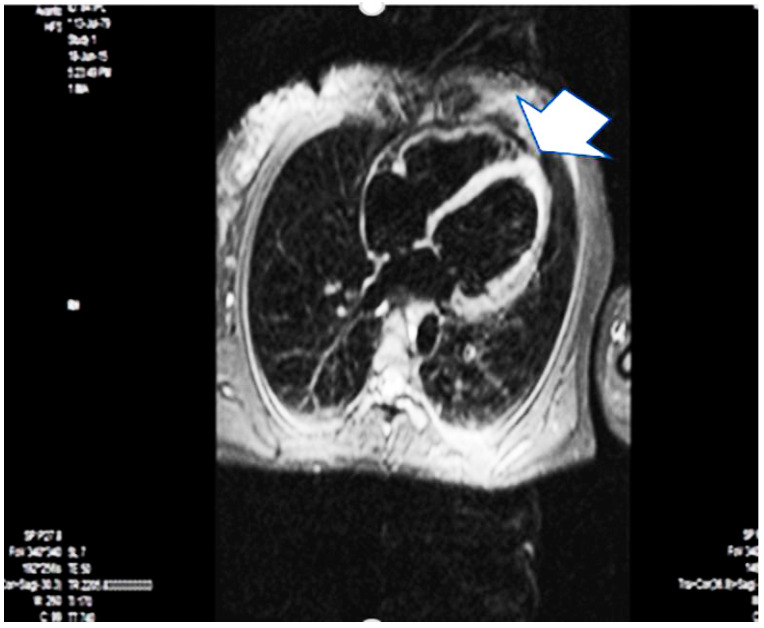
Magnetic resonance imaging–one year later—the arrow points apical fibrosis (scarring). Arrow is apical fibrosis.
